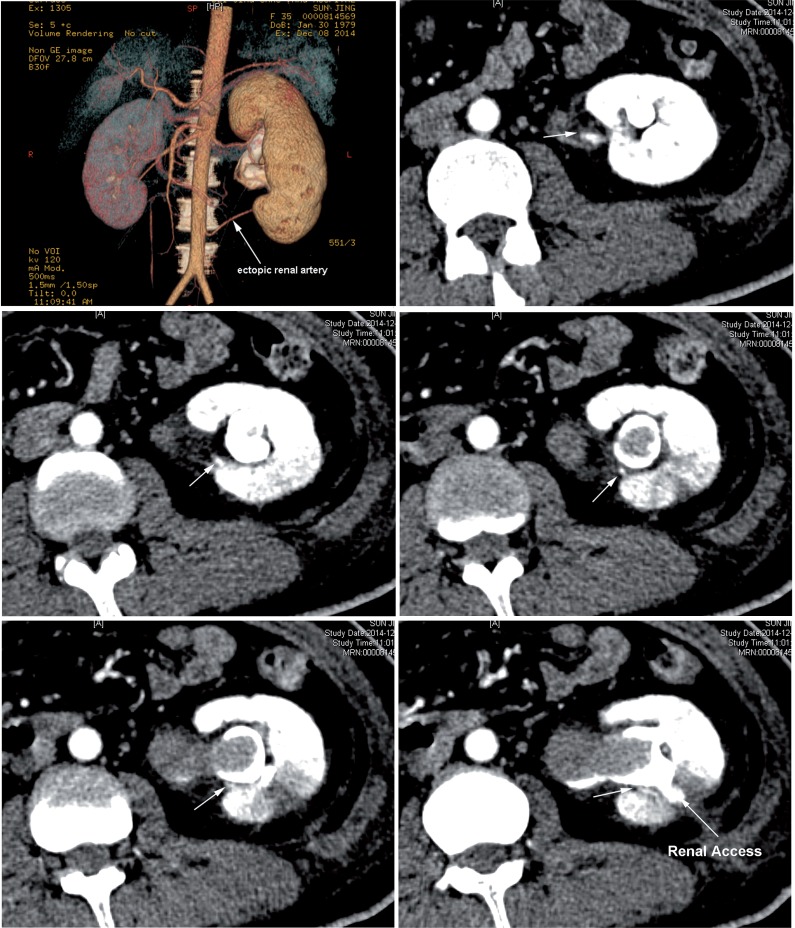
One ectopic renal artery was found in CT angiography of the left renal artery, and a branch of this artery was near the renal access.
